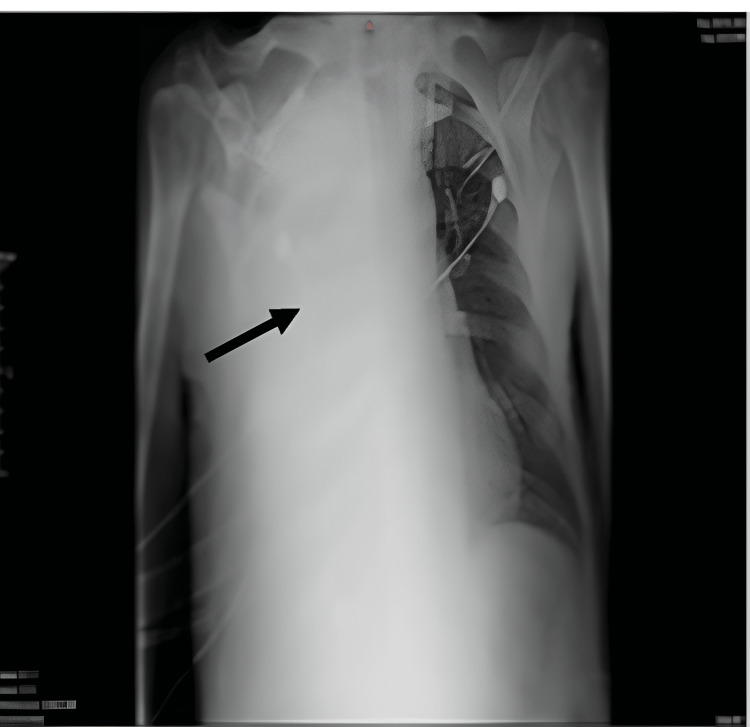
Chest x-ray indicating atelectasis of the right lung. The black arrow indicates complete opacification of the right hemothorax due to right lung atelectasis and the presence of ipsilateral pleural effusion.
